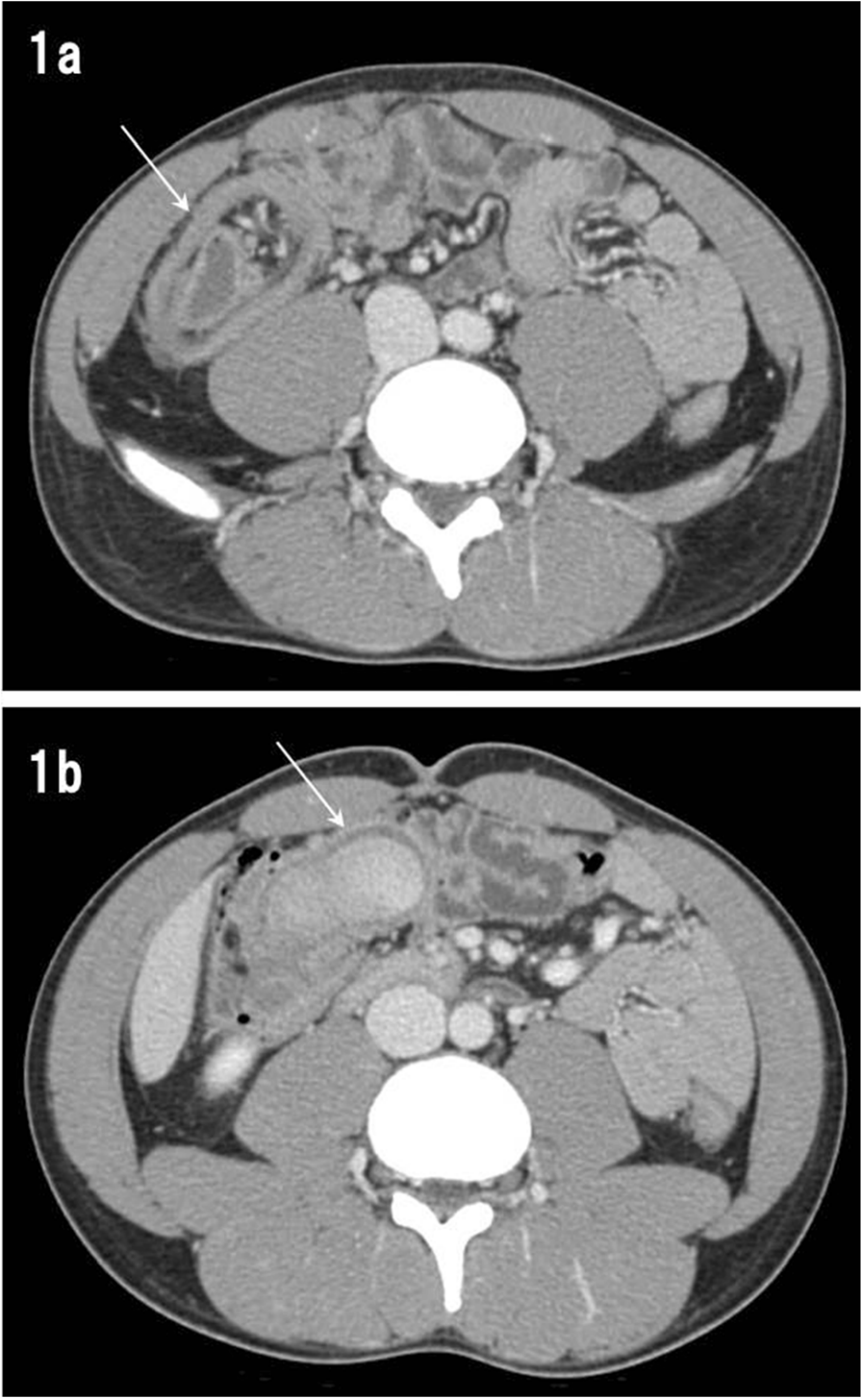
Abdominal computed tomography. a A characteristic “target” sign (white arrow) seen in the right abdomen. b Tumorous mass (white arrow) acting as a leading point for intussusception
